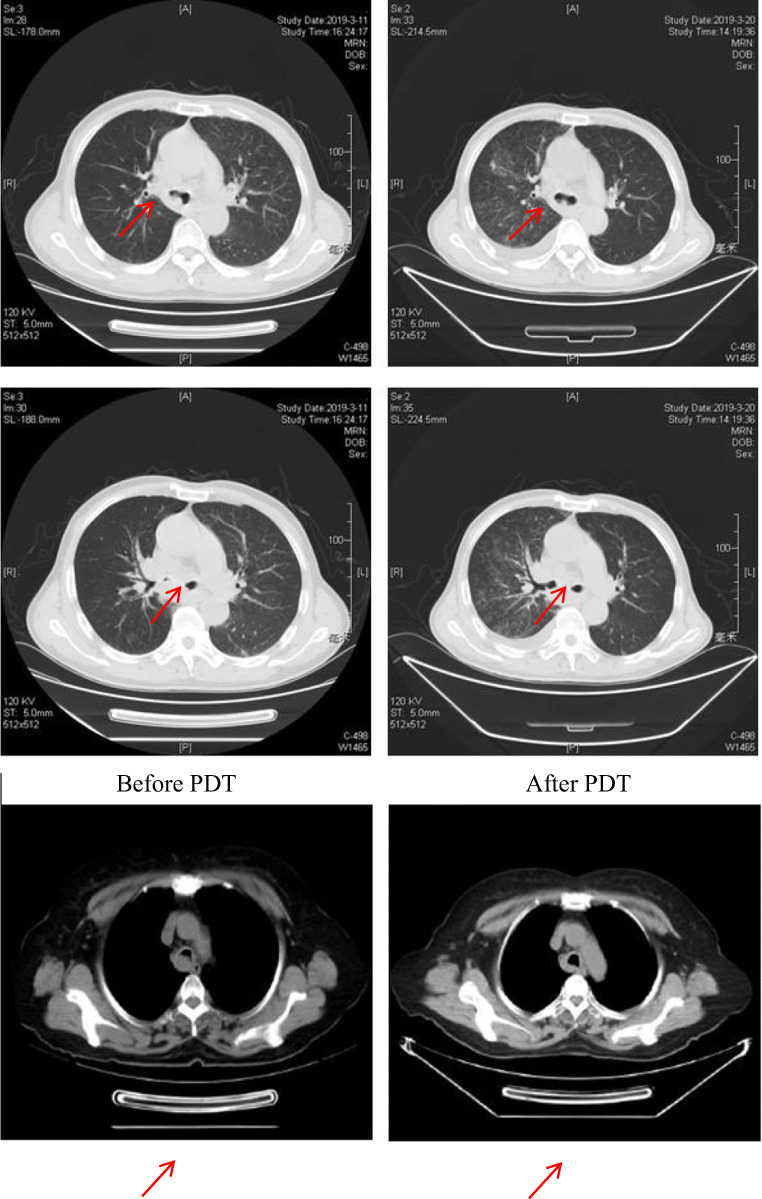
Changes in chest CT 3 months later after PDT. The first column was before the photodynamic treatment, and the second column was the corresponding after treatment
